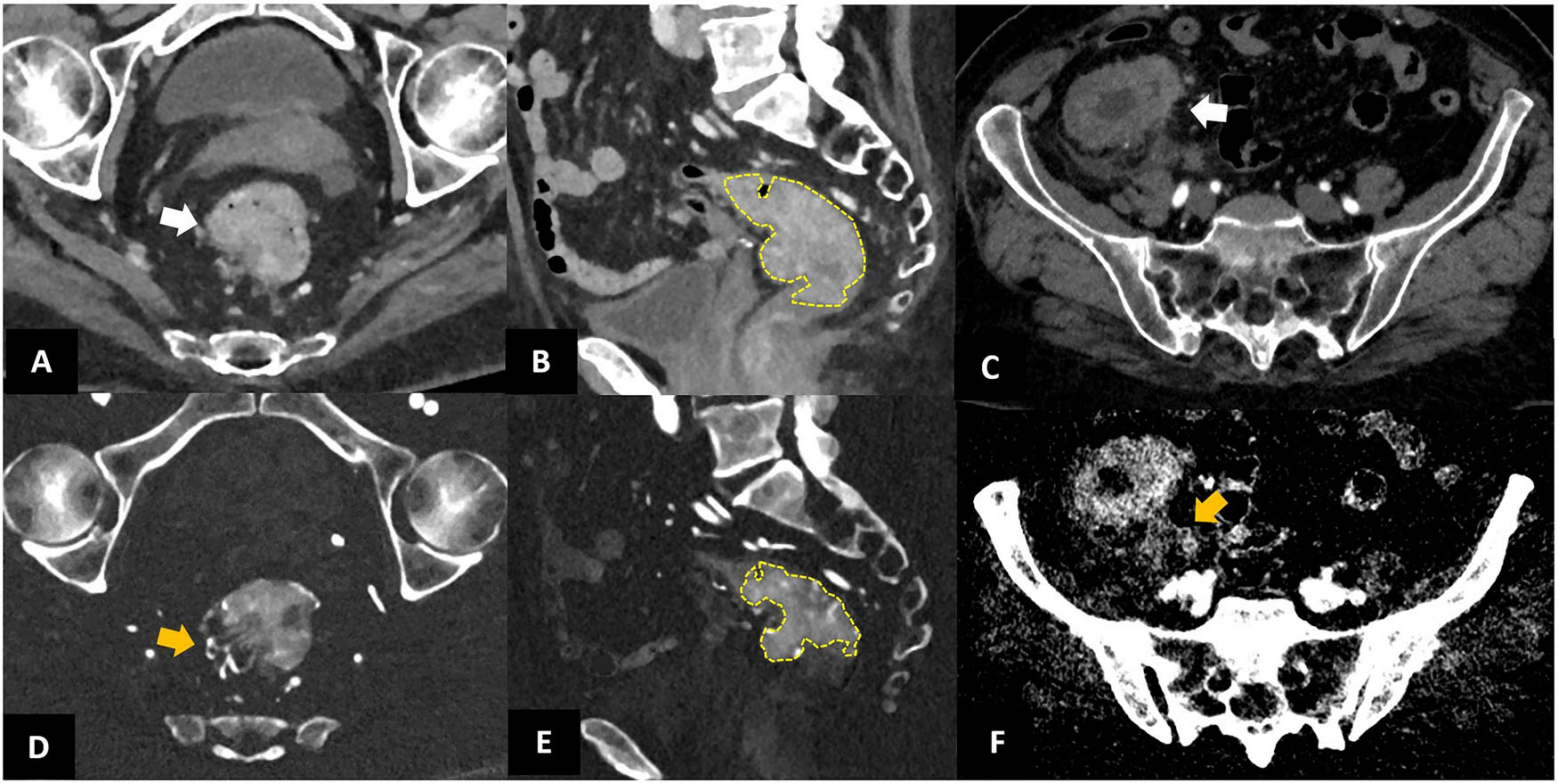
PCCT in colorectal cancer. Axial (A) and sagittal (B) post-contrast scan and the respective iodine maps (D, E) of a 93-year-old submitted to CT for weight loss. PCCT revealed a hypervascular tissue component at the sigmoid-rectal junction. Iodine maps (D, E) clearly identify the implantation base and the pedicle (orange arrow in D) with a more accurate evaluation of its intraluminal and craniocaudal extension (dashed yellow area in E) in comparison to the conventional scan (white arrows in A and dashed yellow area in B), enabling better discrimination of vascularized tissue from fecal content. Axial angiographic PCCT scan (C) and its iodine map (F) of an 86-year-old woman showing severe concentric thickening of the cecal walls, suggestive of neoplasia (white arrow in C), with a maximum thickness of approximately 2 cm and a craniocaudal diameter of about 8.5 cm, involving the ileocecal valve, and associated nodal metastasis. The iodine map improved identification of spiculation into the perivisceral adipose tissue, consistent with disease infiltration, and revealed iodine uptake of the edematous imbibition of the perivisceral adipose tissue surrounding the cecum, suggestive of a lymphangitic process (orange arrow in F)
